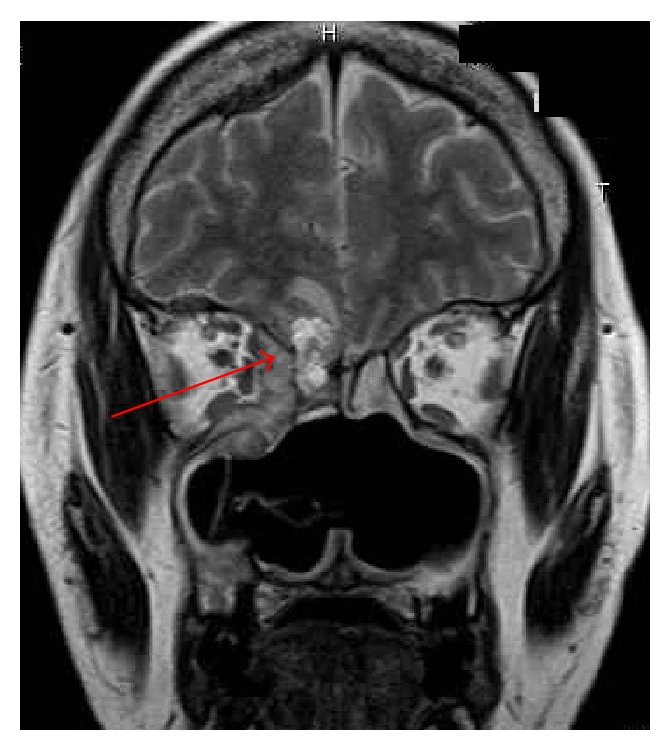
An intracranial invasive lesion (arrow) is shown on this MRI image.
